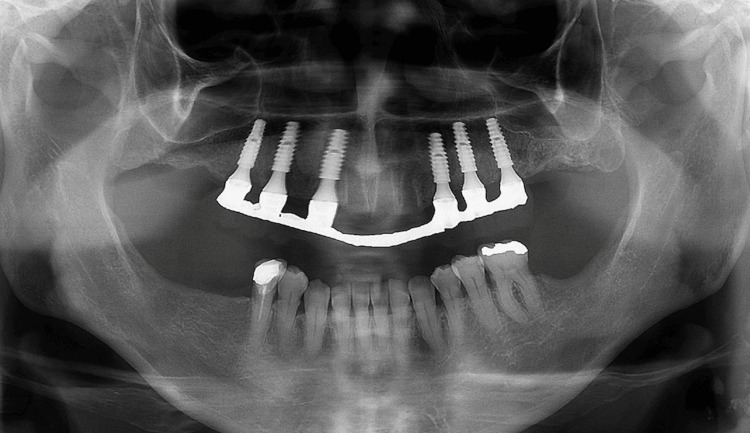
Twelve-month panoramic radiograph with the final prosthesis installed
